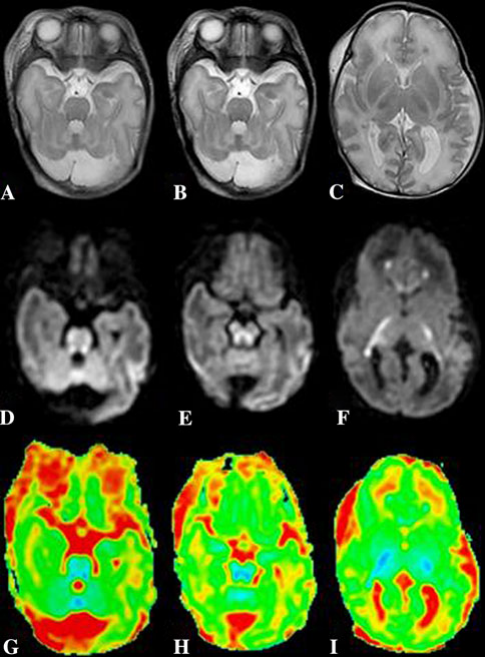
MR images. Axial fast spin-echo T2-weighted MR images show high signal intensity in the middle cerebellar peduncles (a), the dorsal brain stem (b), and the in the posterior limbs of the internal capsules (c) suggesting abnormal myelin. d, e, f Diffusion-weighted MR image demonstrates high signal intensity in these areas. g, h, i ADC map shows decreased ADC values in these areas consistent with restricted diffusion
